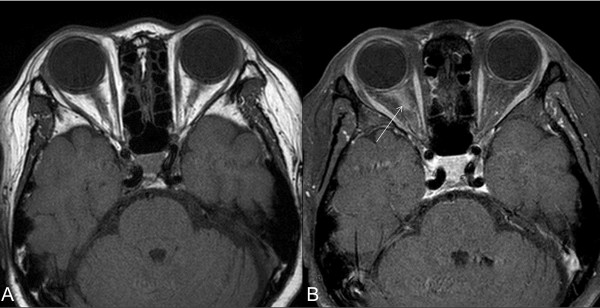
Brain magnetic resonance imaging 1 week after clinical onset of visual loss in the right eye. Axial fat saturated T1-weighted imaging (T1WI) (A) and contrast-enhanced T1WI (B) shows enhancement of the intraorbital portion of the right optic nerve (white arrow).
